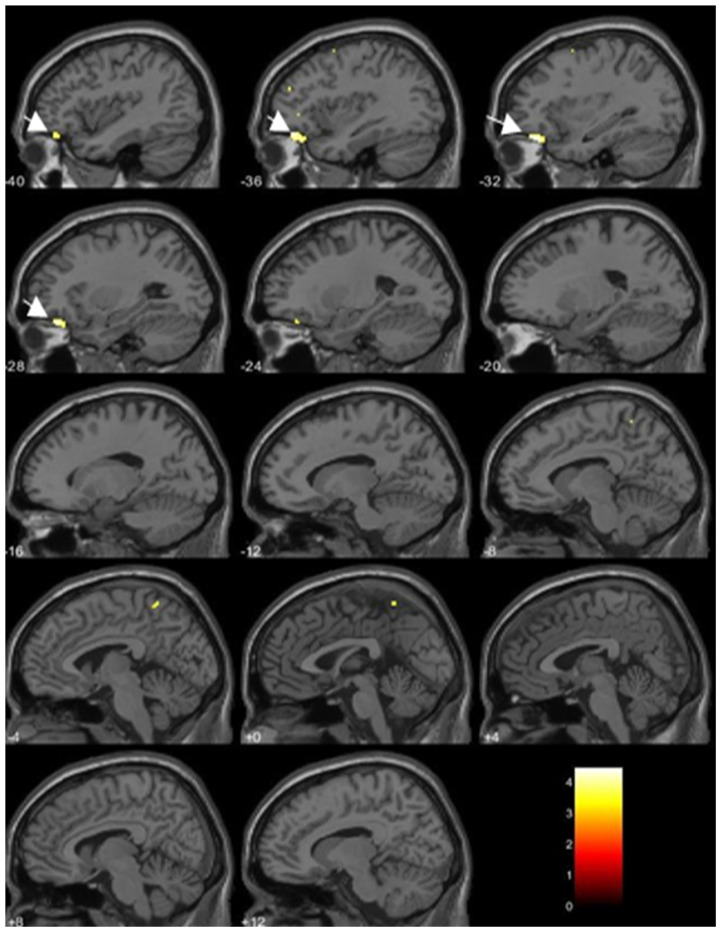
Brain regions associated with perinatal dioxin exposure (the left inferior frontal gyrus pars orbitalis indicated by yellow color and arrows on sagittal MRI slices). The volume was significantly lower in men with estimated perinatal dioxin exposure than in men without perinatal exposure (FDR-corrected at *p* < 0.05). Each value below each brain slice indicates each value in MNI x-coordinates.
